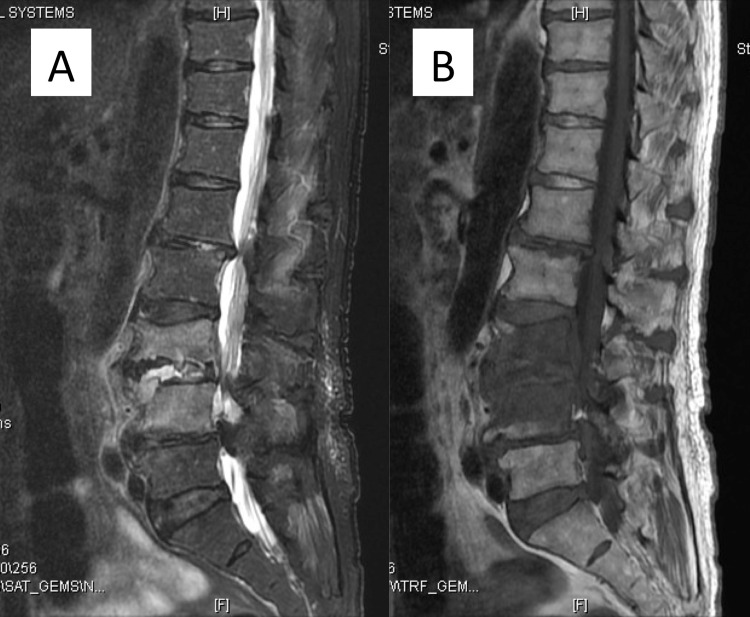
Secondary images obtained with magnetic resonance imaging. (A) Sagittal T2-weighted fat-suppressed image. (B) Sagittal T1-weighted image. Fluid collection in the intervertebral disc space and diffuse signal changes in the L3 and L4 vertebral bodies with destructive changes were observed.
